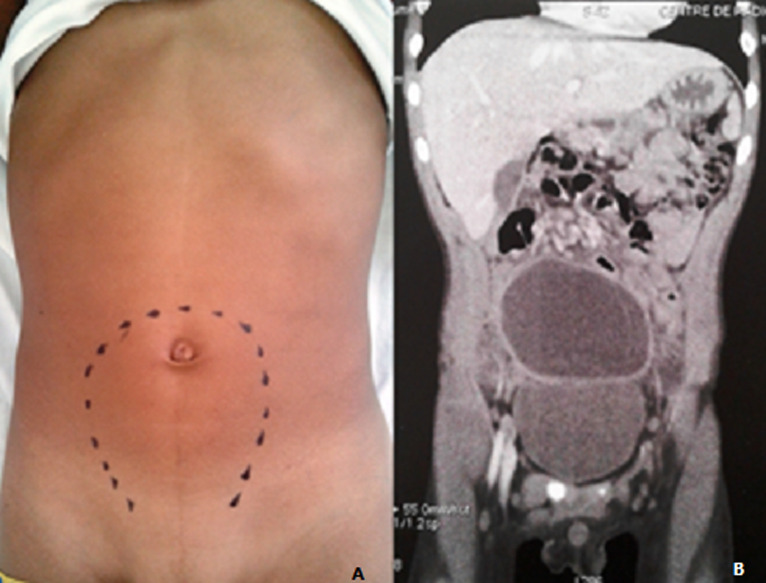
abdominal examination indicating the site of the mass and the contrast enhanced CT image showing a cystic mass above the bladder (A,B)
